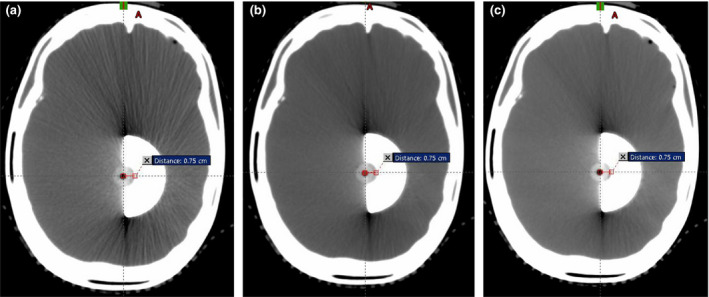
Axial CT slice through the center of the kyphoplasty object, imaged at (a) 90 kV_p_, (b) 120 kV_p_, and (c) 140 kV_p_.
